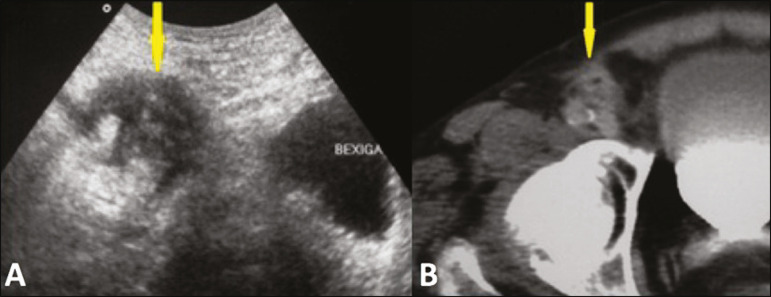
A: Axial ultrasound showing an ill-defined, hypoechoic, heterogeneous area in the inguinal canal. B: Axial CT of the same patient, showing a thickened appendix in the inguinal canal.
